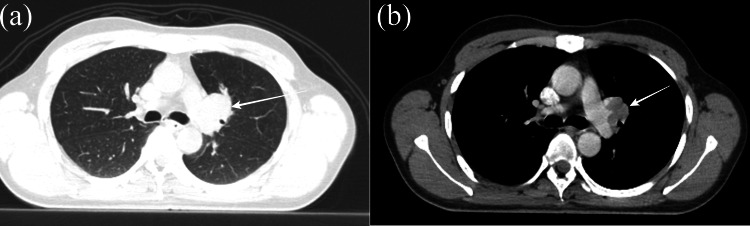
(a) Axial lung window (b) axial mediastinal window of CT scan displays a perihilar mass (white arrow) abutting the left main bronchus and left pulmonary artery
